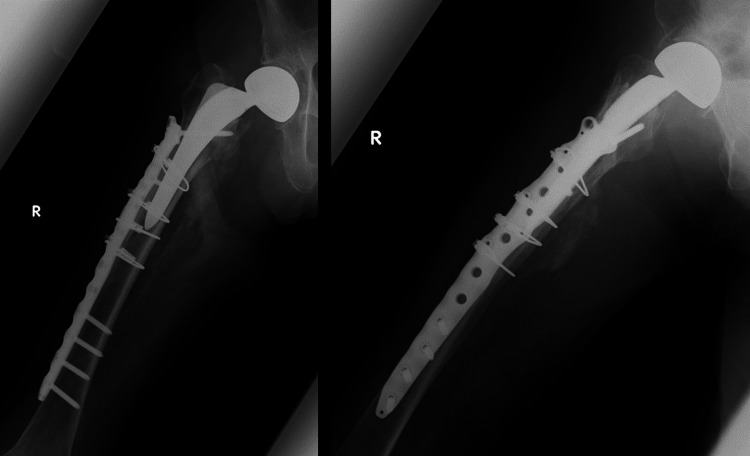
Postoperative radiographs of case 1 ORIF with plate fixation and cerclage wiring was performed. ORIF: open reduction and internal fixation.
